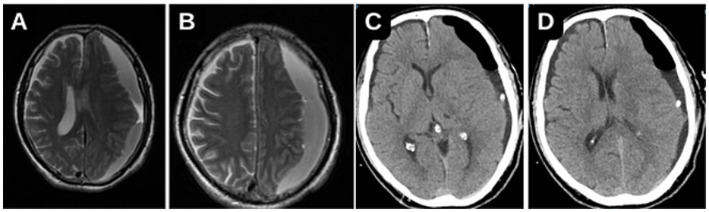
Radiological presentation and surgical outcome for case 1. (A,B) Preoperative imaging indicating significant layering and separation within the complex septated chronic subdural hematoma, causing associated mass effect. (C,D) The corresponding postoperative CT scan demonstrates 96% hematoma volume reduction and symmetrical brain re-expansion of the subdural collection.
